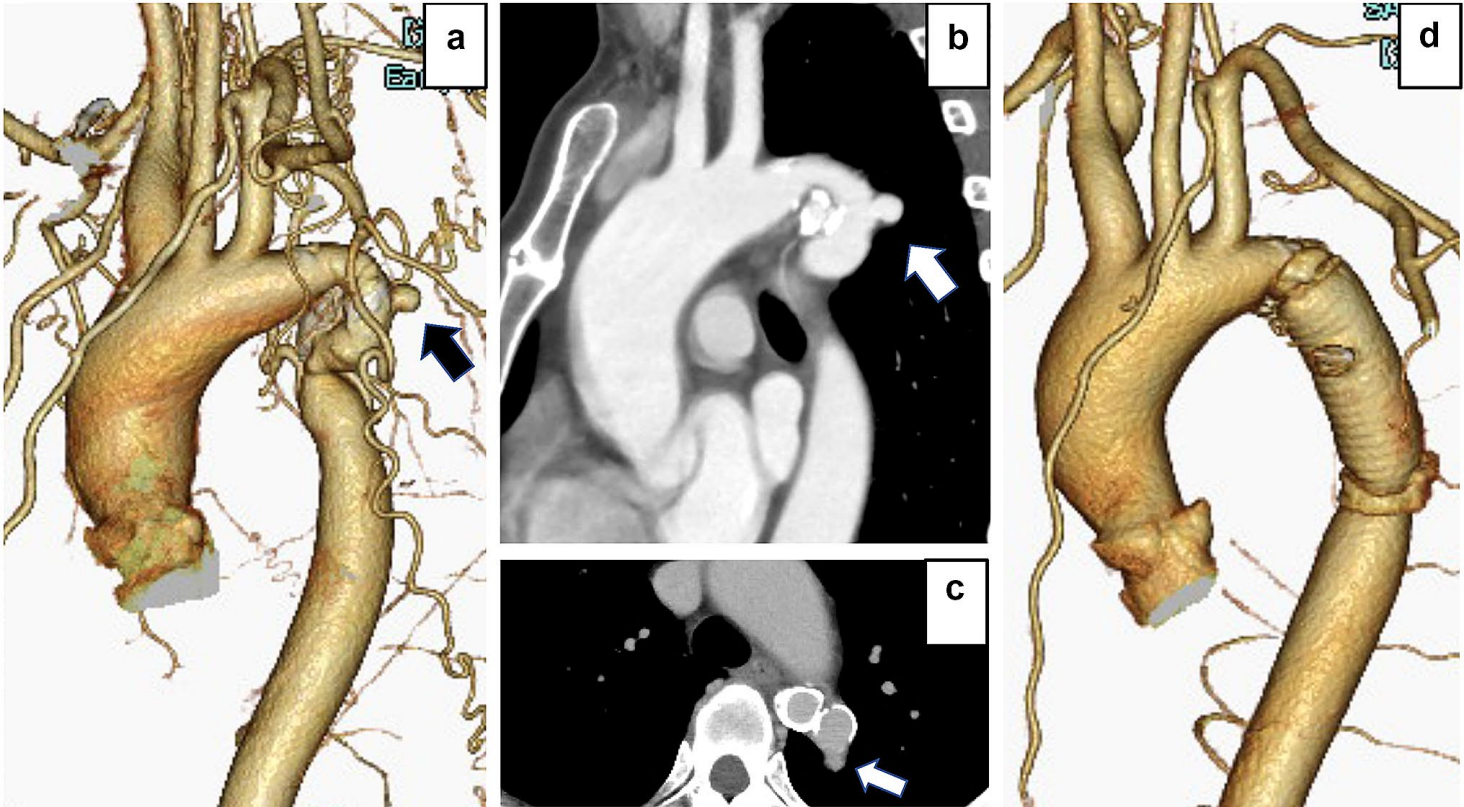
a Preoperative contrast-enhanced CT scan: a saccular aortic aneurysm is observed at the site of aortic coarctation (arrow). Collateral circulation appears well developed. b Preoperative contrast-enhanced CT scan, sagittal section: a saccular aortic aneurysm protruding dorsally is observed. The aneurysm wall appears very thin (arrow). c Preoperative contrast-enhanced CT scan, axial section: a dorsally protruding saccular aortic aneurysm is observed, the arterial wall other than the aneurysm appears highly calcified. d Postoperative contrast-enhanced CT scan: the stenosis was completely resected
